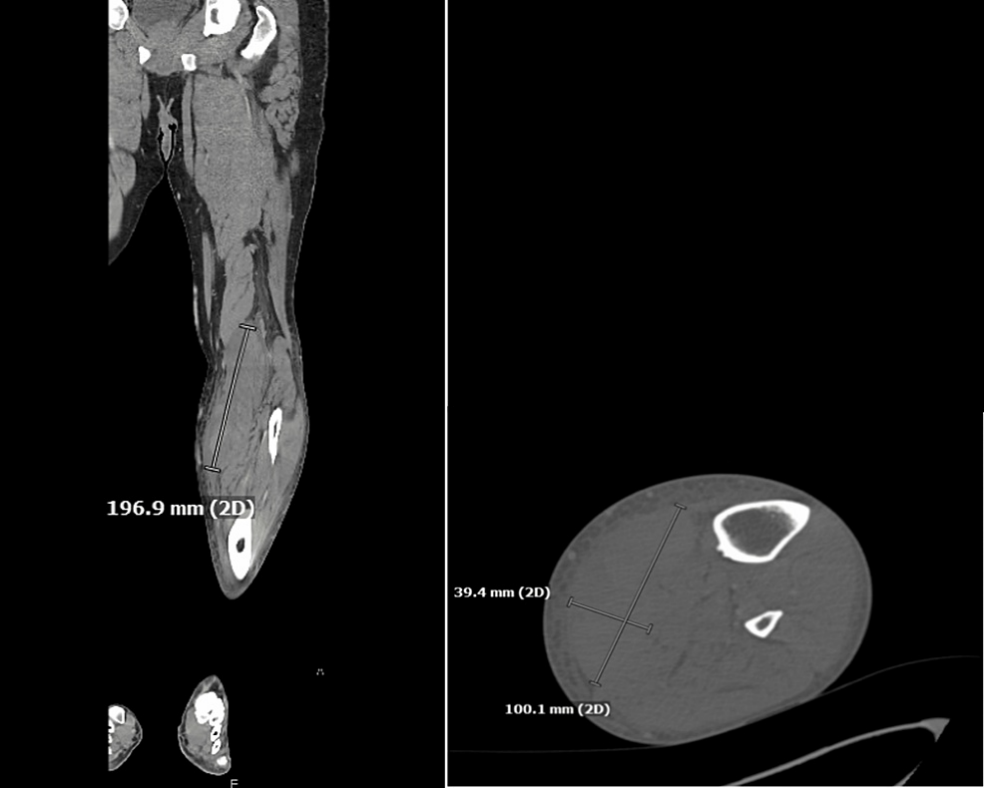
Coronal and axial CT of the left lower extremity with intravenous contrast demonstrates a hematoma measuring 100.1 mm anterior-posteriorly × 39.4 mm transversely × 196.9 mm cranio-caudally.
